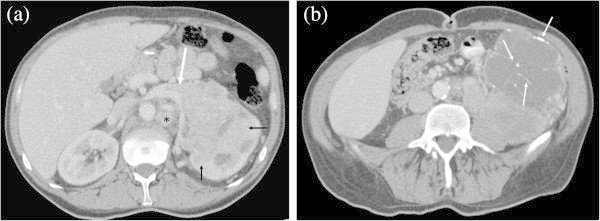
A 43-year-old male with Fuhrman grade 4 ccRCC showing infiltrative growth. a: Transverse contrast-enhanced CT shows a large heterogenous mass in the left kidney. The border between the tumor and normal kidney is ill-defined, representing infiltrative tumor growth (small arrows). A large arrow and asterisk show tumor thrombus in the left renal vein and left paraaortic lymphadenopathy, respectively. b: Transverse contrast-enhanced CT below the level of Figure
